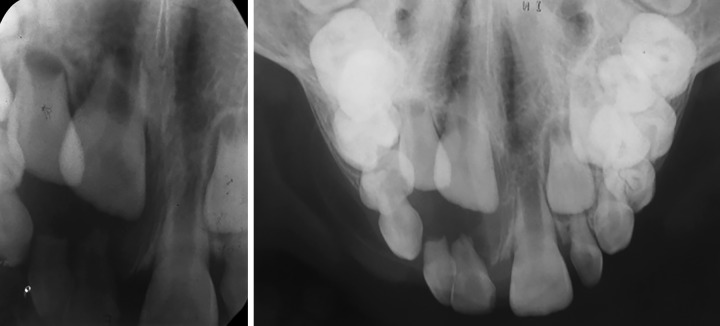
Intraoral periapical and occlusal radiograph
